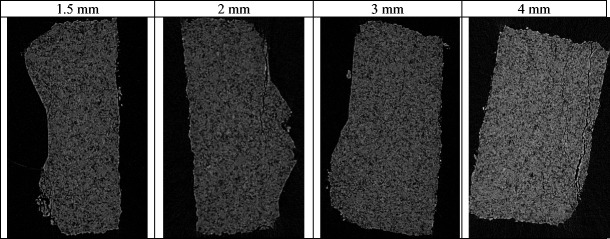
X-ray images of ribbon produced at different roller gap
